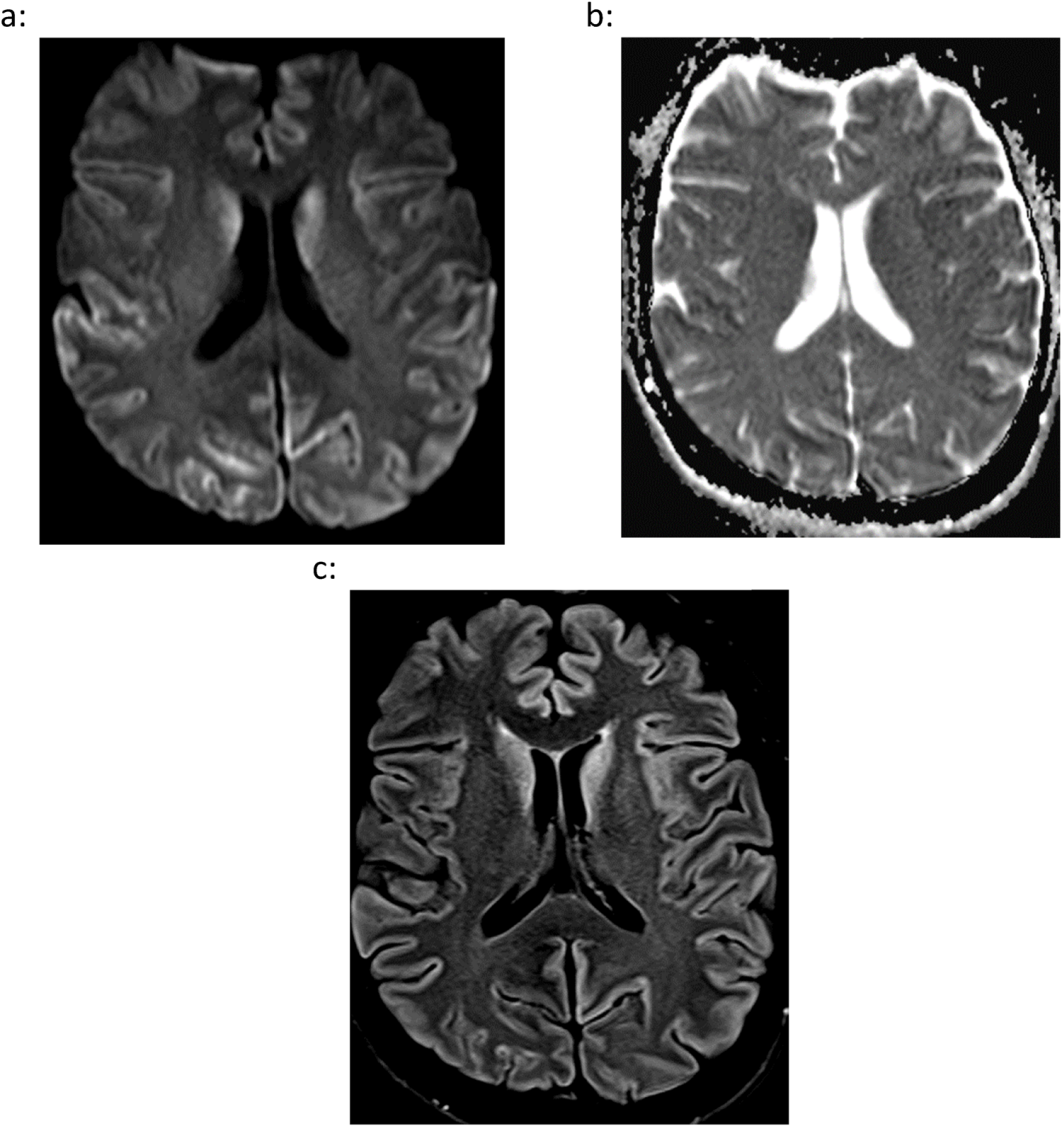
Patient with sCJD (female, 53 years old) initially presenting with progressive cognitive decline leading to akinetic mutism with pyramidal and cerebellar features. No positive family history concerning neurodegenerative diseases, no corneal transplantation. Normal values for glucose, protein, and immunoglobulin G in CSF, normal blood values. Elevation of 14-3-3 protein in CSF and positive reaction in RT-QuIC. MRI showed nearly symmetrical high signals in the parietal, occipital, and temporal cortex and caudate nucleus on diffusion-weighted imaging, DWI (a). The corresponding map for the apparent diffusion coefficient, ADC, presented low values in these regions, which is suggestive of a restriction of the diffusion (b). In the fluid-attenuated inversion recovery sequence, FLAIR, these regions showed a variably hyperintense signal (c). (a) DWI, diffusion-weighted imaging (*b*-value: 1,000 s/mm²), (b) ADC, apparent diffusion coefficient, and (c) FLAIR, fluid-attenuated inversion recovery sequence.
